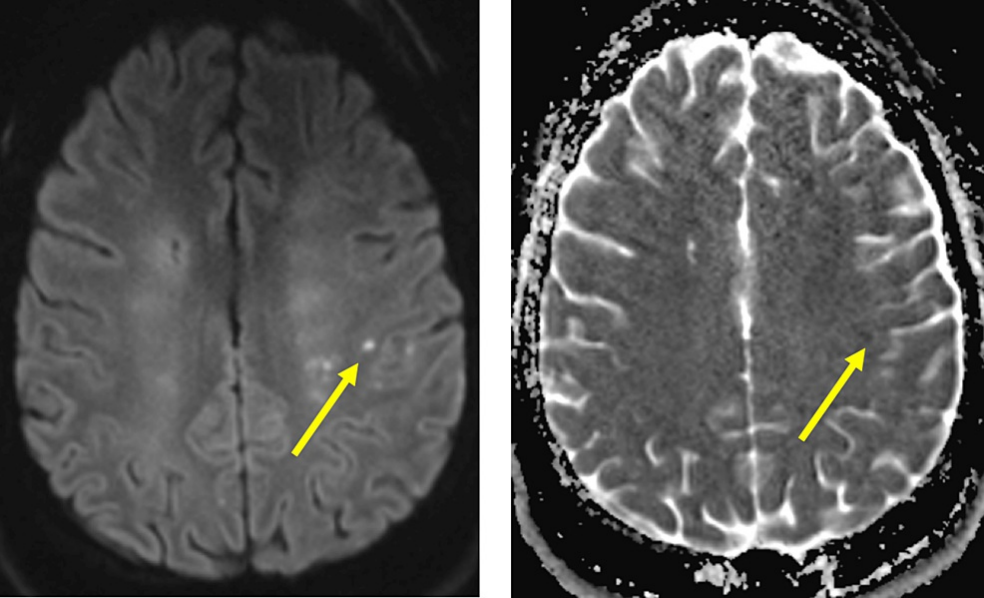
Small focus of T2 hyperintensity in the subcortical white matter in the left posterior frontal lobe (yellow arrows) which shows restricted diffusion on DWI/ADC mapping suggestive of an acute ischemic event, possibly embolic in etiology DWI/ADC: diffusion weight imaging/apparent diffusion coefficient.
